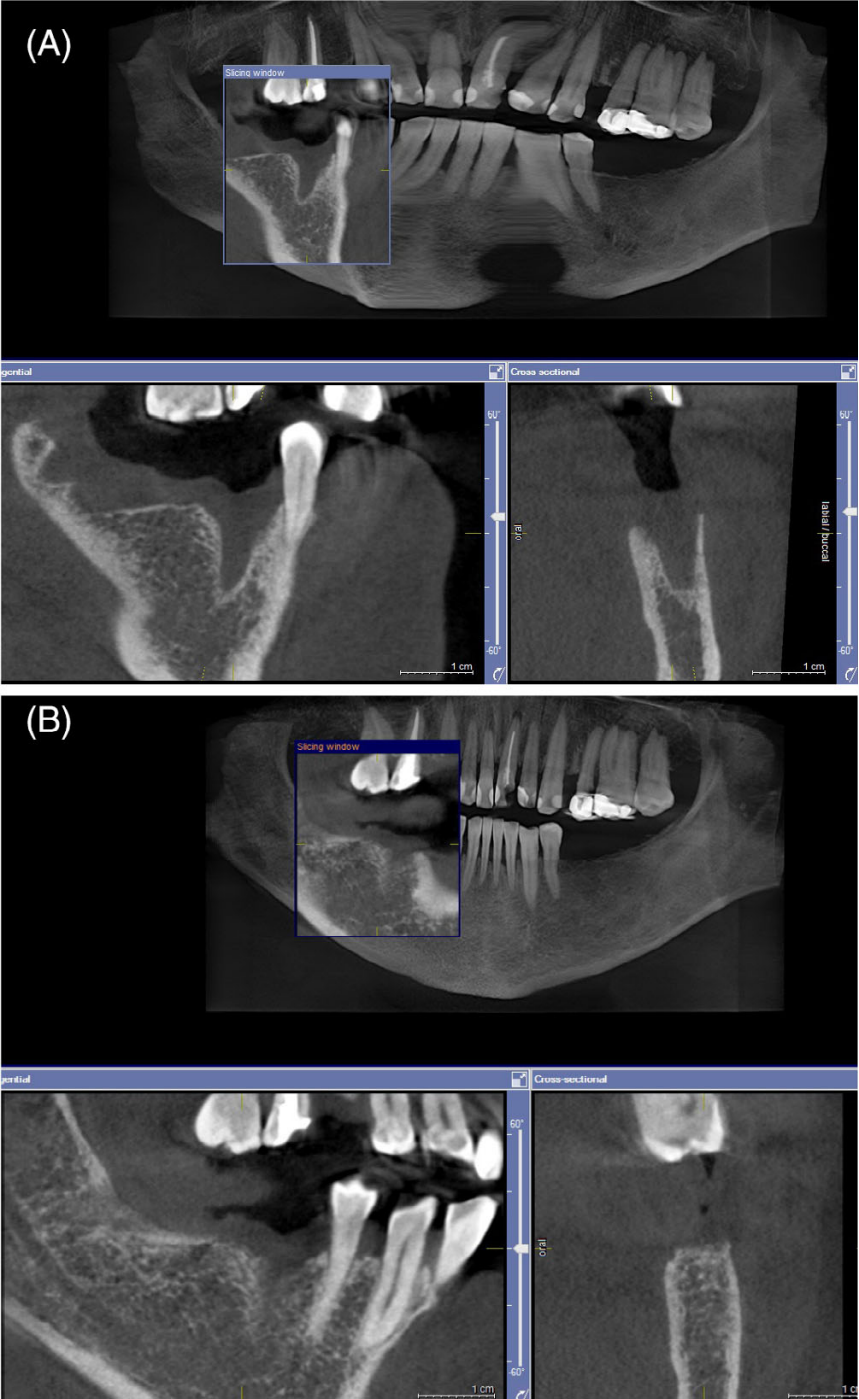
Female, 56 years old, extraction of 45 and 47 due to chronic periodontitis. Laser post‐extraction procedure was used. CBCT scan 1 day after extraction (A) and at for month follow up (B)
